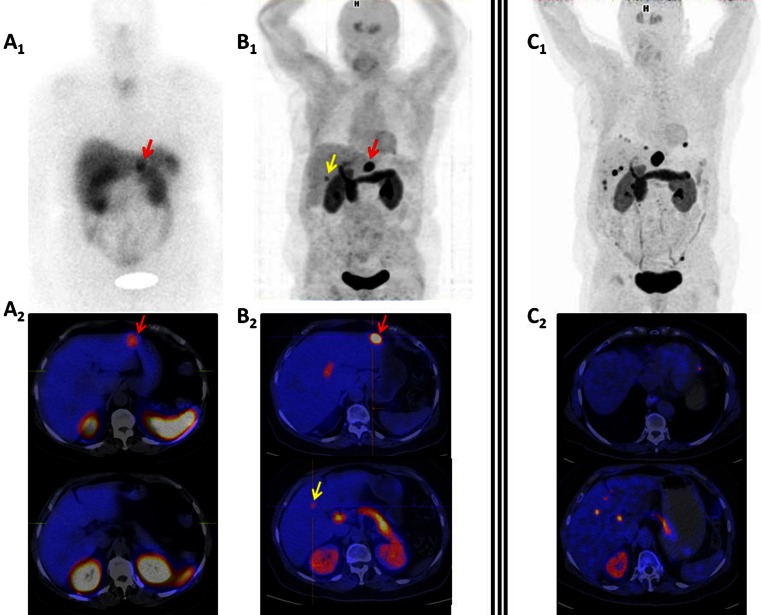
SRS using ^111^In-pentetreotide (*a*) and ^18^F-FDOPA PET/CT (*b*) performed 1 week apart in a patient with a well-differentiated metastatic ileal NET. Planar scintigraphy (*a1*) shows an uncertain liver lesion (*red arrow*), clearly located in segment II of the liver in the SPECT/CT fusion image (*a2*). Whole-body PET/CT image using ^18^F-FDOPA (*b1* and *b2*) shows the same liver metastasis (*red arrow*) but also detects an additional liver lesion in segment V (*yellow arrow*). Follow-up ^18^F-FDOPA PET/CT (*c1* and *c2*) performed after 12 cycles of octreotide therapy identifies multiple hepatic and peritoneal implants (both abdominal and subdiaphragmatic costophrenic angle) reflecting tumor progression
